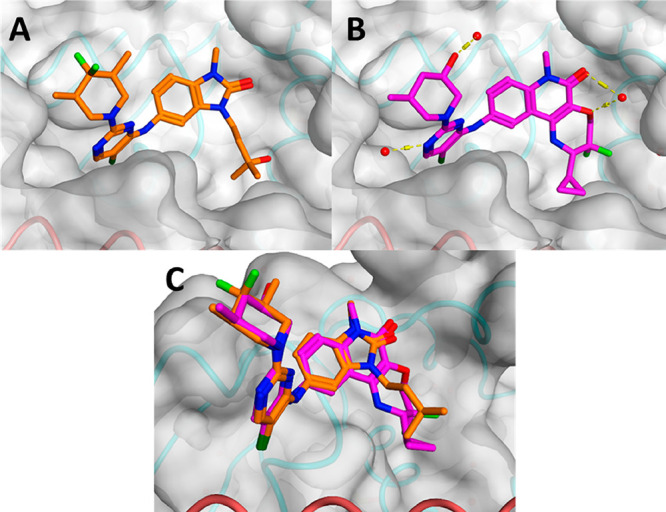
(A) X-ray structure of the BCL6 BTB domain with the bound
